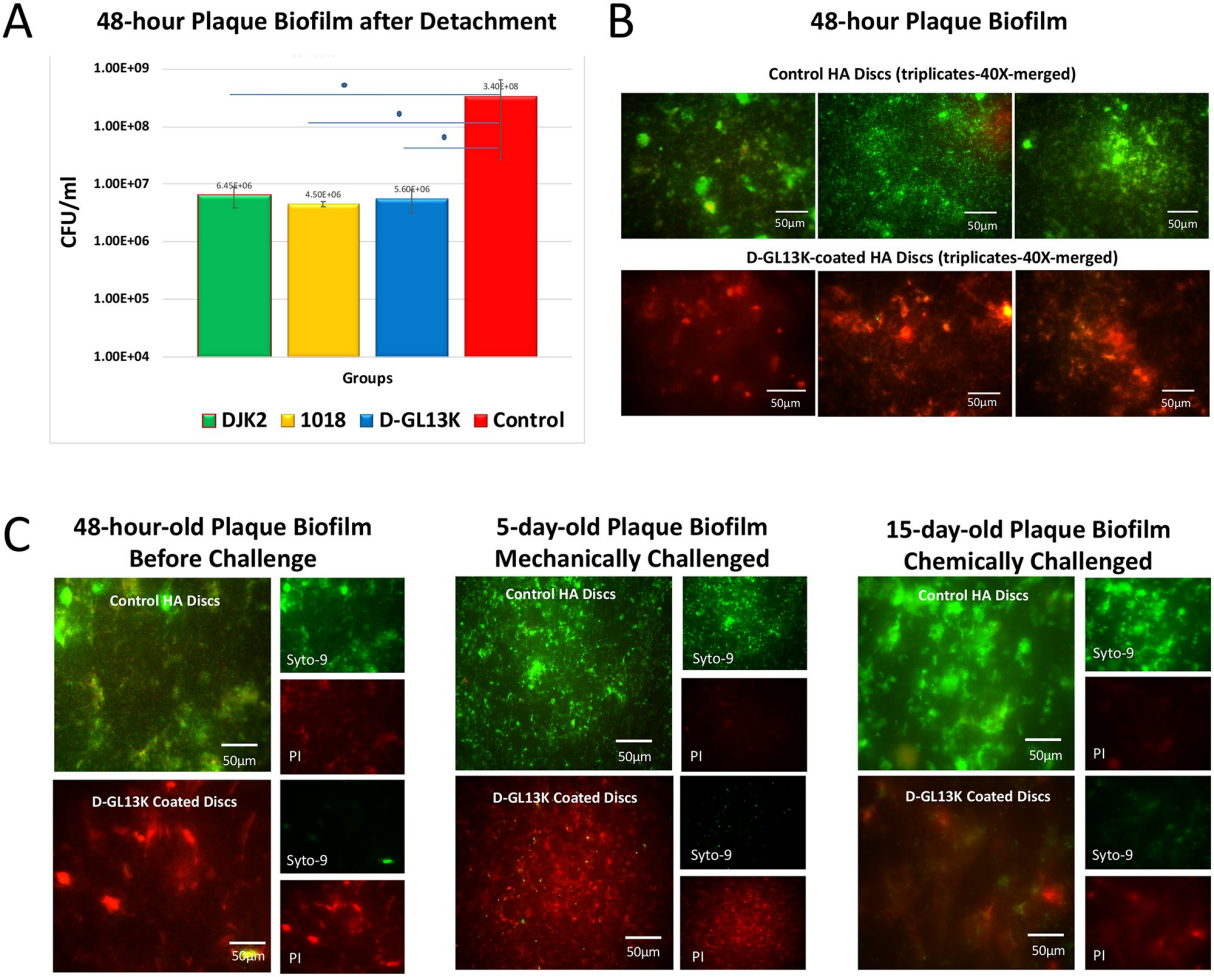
Antibiofilm effect of D-GL13K, DJK2, and 1018 coated HA discs on dental plaque biofilms. A) Cell death quantification by counting colony forming units (CFU) for detached bacteria biofilms from HA discs coated with all AAMPs. Control group is 32% phosphoric acid-etched non-coated HA discs. N = 3. B) Merged live (green) and dead (red) bacteria viability assay images of 48-hour plaque biofilms grown on HA discs with (bottom image) and without (top image) D-GL13K coatings. C) Live and dead viability assay images of 48-hour (left), 5-day (middle), and 15-day-old (right) plaque biofilms grown on HA discs with (bottom images) and without (top images) D-GL13K coating. Middle panel shows regrown biofilms for 5 days after 45 min of ultrasonication (mechanically challenged D-GL13K coating). Right panel shows regrown biofilms for 15 days after 45 min immersion in 30% acetic acid (chemically challenged D-GL13K coating). Merged images are on the left side of each group and separated live (top)/dead (bottom) bacteria images are on the right side of each group.
